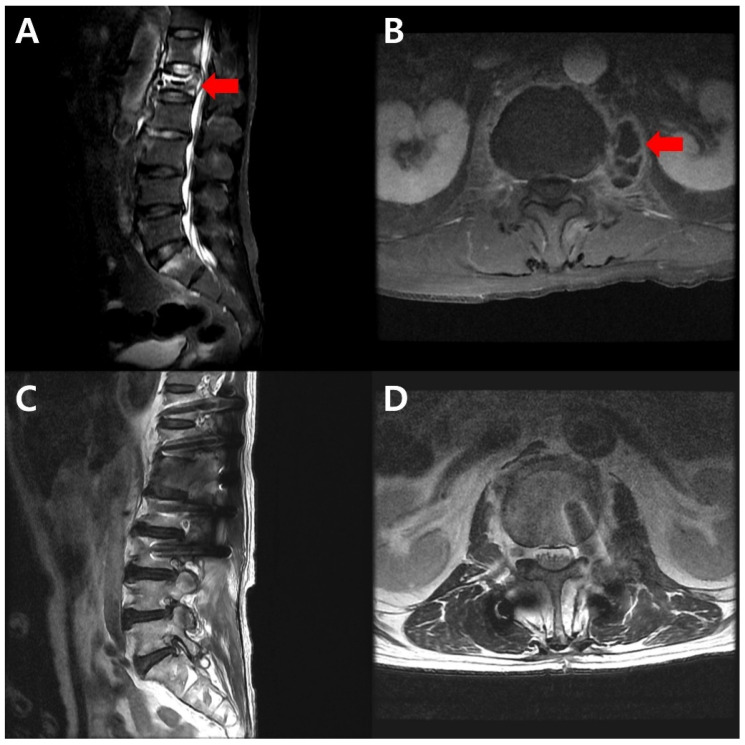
Spinal MRI findings with thoracolumbar tuberculous spondylitis before and at the end of the treatment. (A) A T2-weighted sagittal sequence in the baseline spine MRI revealed destruction of the vertebral bodies, particularly at L1, with contrast enhancement (red arrow). (B) A T1-weighted fat-suppressed axial sequence in the baseline spine MRI demonstrated a left psoas abscess (red arrow). (C) A T2-weighted sagittal sequence in the follow-up MRI obtained at the end of the therapy demonstrated marked resolution of the inflammatory changes in the vertebral body. (D) A T2-weighted axial sequence in the follow-up MRI obtained at the end of the therapy demonstrated improvement of the left psoas abscess.
